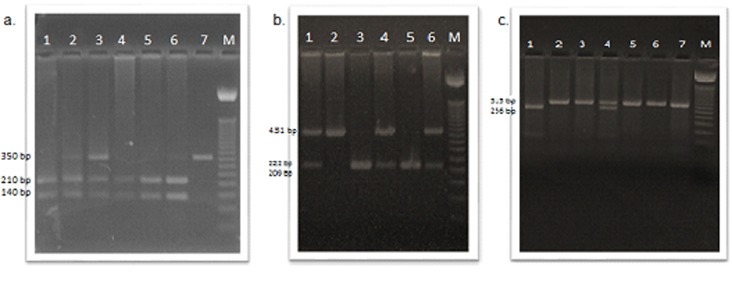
Genotyping for rs320, rs285 y rs328 polymorphisms in *lipoprotein lipase* gene. Restriction fragments length polymorphisms (RFLP) on 2% agarose gel. a. rs320; lane 1, 4, 5, 6, TT genotype; lane 2, 3, GT genotype; lane 7, GG genotype; b. rs285; lane 1, 4, 6, CT genotype; lane 2, CC genotype; lane 3,5, TT genotype. c. rs328; lane 1, GG genotype; lane 2, 3, 5, 6, 7, CC genotype; lane 4 CG genotype; M, DNA 50 bp marker*.*
